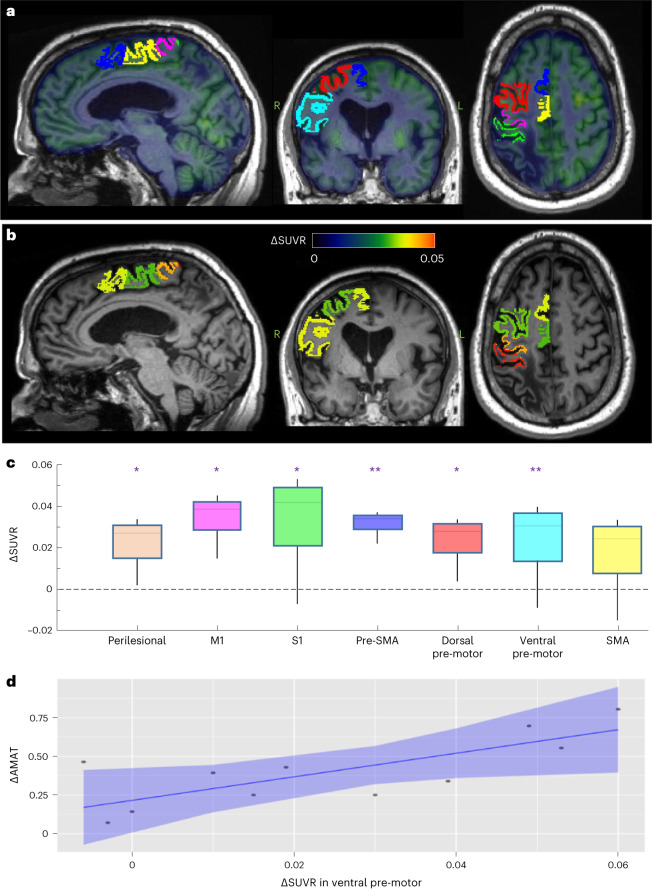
Change in brain metabolism associated with DN-DBS combined with rehabilitation and relationship to treatment-related changes in arm function. a, Magnetic resonance imaging (MRI) overlayed by PET from a 58-year-old male participant with a right hemisphere stroke lesion. The ipsilesional motor-associated cortical regions are identified as: primary motor (M1), fuchsia; primary somatosensory (S1), green; pre-supplementary area (pre-SMA), blue; dorsal pre-motor, red; ventral pre-motor, cyan; SMA, yellow. b, In the same participant shown in a, the average ΔSUVR adjusted for uptake time in response to DN-DBS combined with rehabilitation in the ipsilesional motor-associated cortical regions is shown. c, The change in brain metabolism in response to DN-DBS combined with rehabilitation in perilesional and ipsilesional motor-associated cortical regions for all participants. Linear mixed-effects models were used to test for significant change in the mean SUVR between rehab-only and rehab-carryover phases of the trial, with time point and ^18^F-FDG uptake time (that is, time between 18-FDG injection and start of PET scan) as fixed effects, with participant as the random effect intercept, and applying the default unstructured covariance structure. The box is the median and interquartile range, the whiskers are the maximum and minimum of the average ΔSUVR adjusted for uptake time, *n* = 11 participants. Two-sided *P* values with no adjustments for multiple comparison: perilesional *P* = 0.007, M1 *P* = 0.007, S1 *P* = 0.002, pre-SMA *P* = 0.0002, dorsal pre-motor *P* = 0.004, ventral pre-motor *P* = 0.0006, SMA *P* = 0.08. P* < 0.05, *P* < 0.001. d, A significant association was identified between change in brain metabolism and change in arm function (as measured by ΔAMAT) in ipsilesional ventral pre-motor cortex. The line is the linear fit adjusting for uptake time and the error bands are the confidence intervals. *F*-statistic *P* = 0.04. Leave-one-out cross-validation: root mean squared error = 0.197; *R*^2^ = 0.247; mean absolute error = 0.176.
